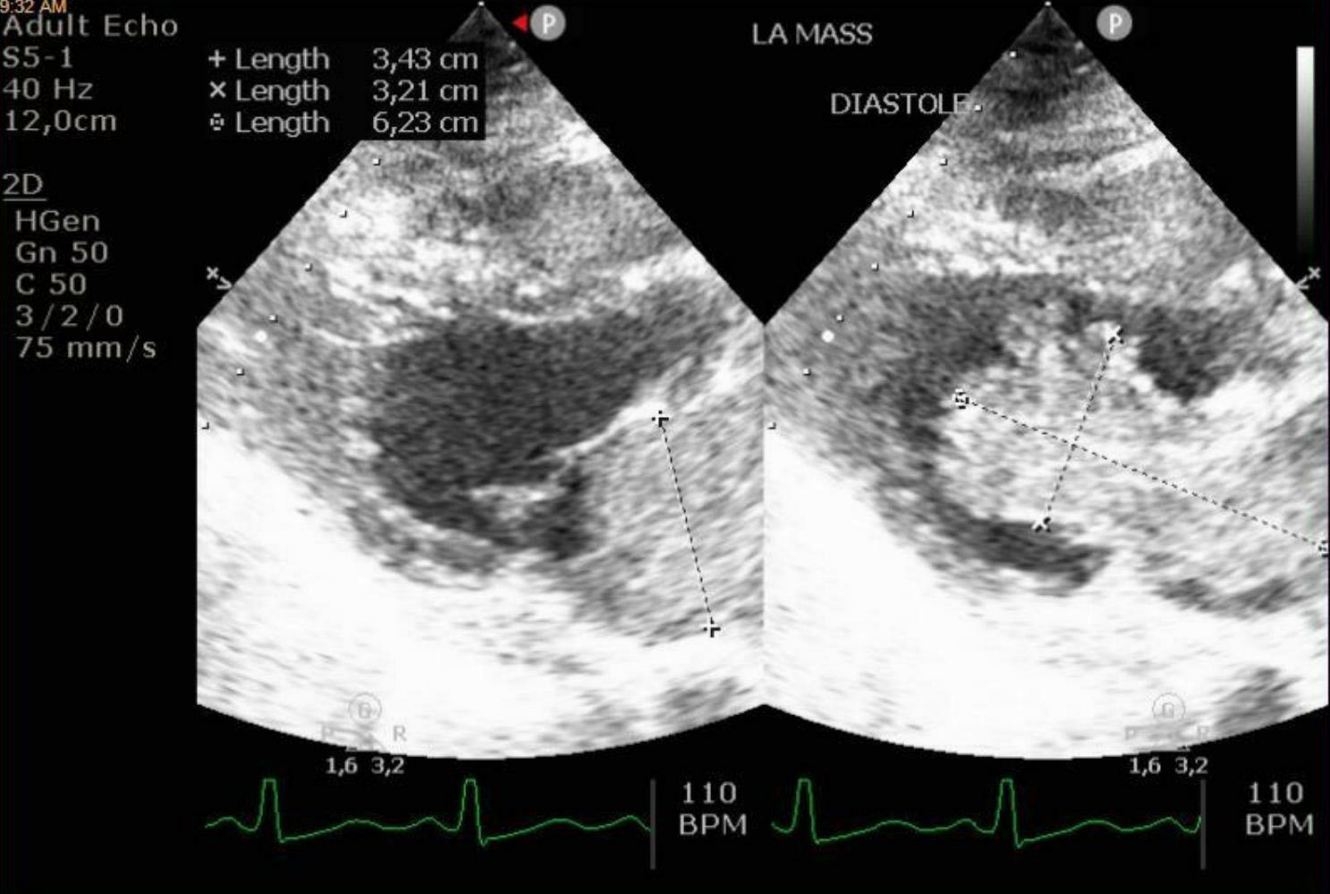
Two-dimensional transthoracic echocardiogram of the left atrial mass.
